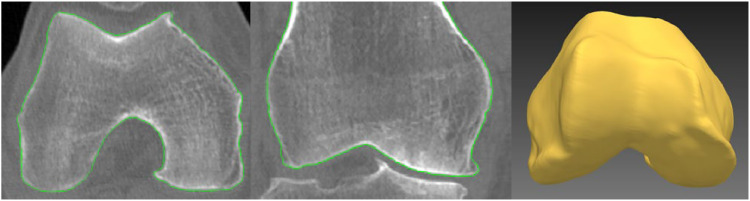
Example of 3D auto segmentation of the distal femur in a CT image from the IMI-APPROACH dataset. Left: transverse plane, middle: coronal plane, and right: 3D rendered surface.
